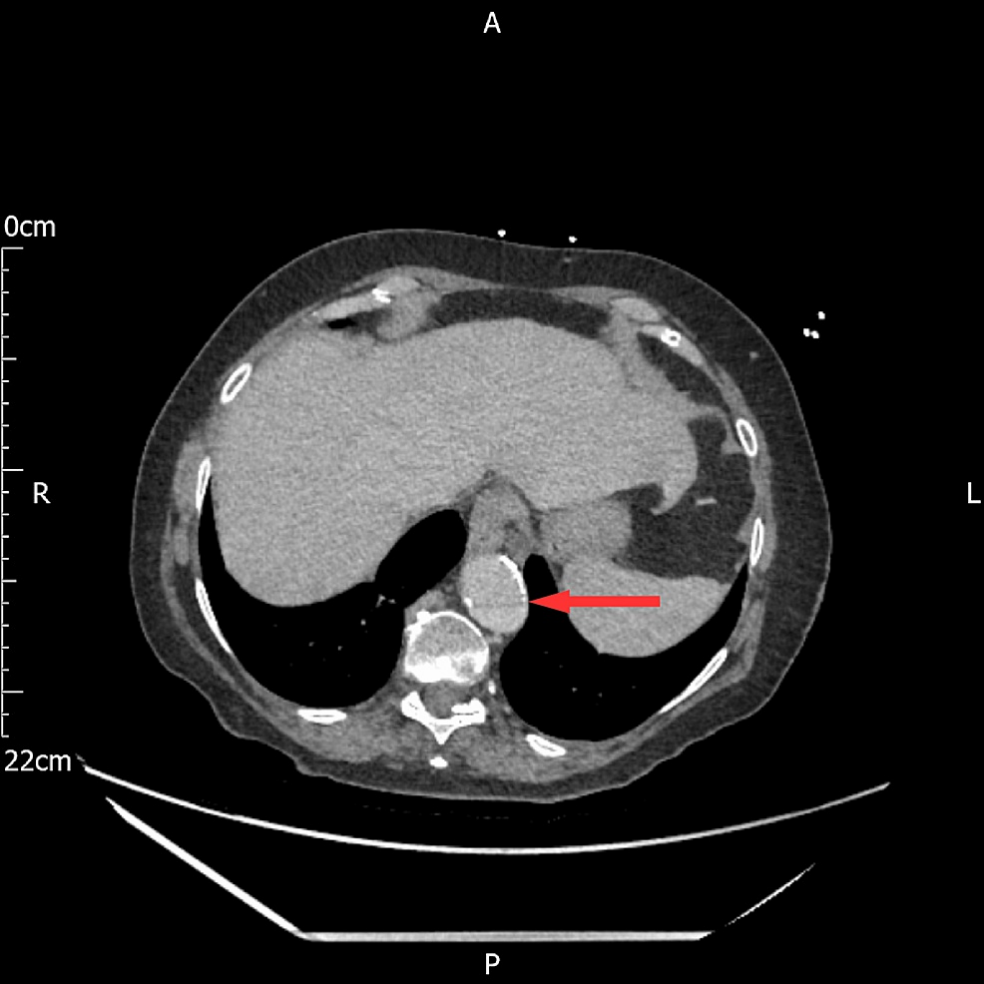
Portal venous phase CT AP showing aortic dissection at the T 10 level CT AP; computed tomography of abdomen and pelvis, T; thoracic
